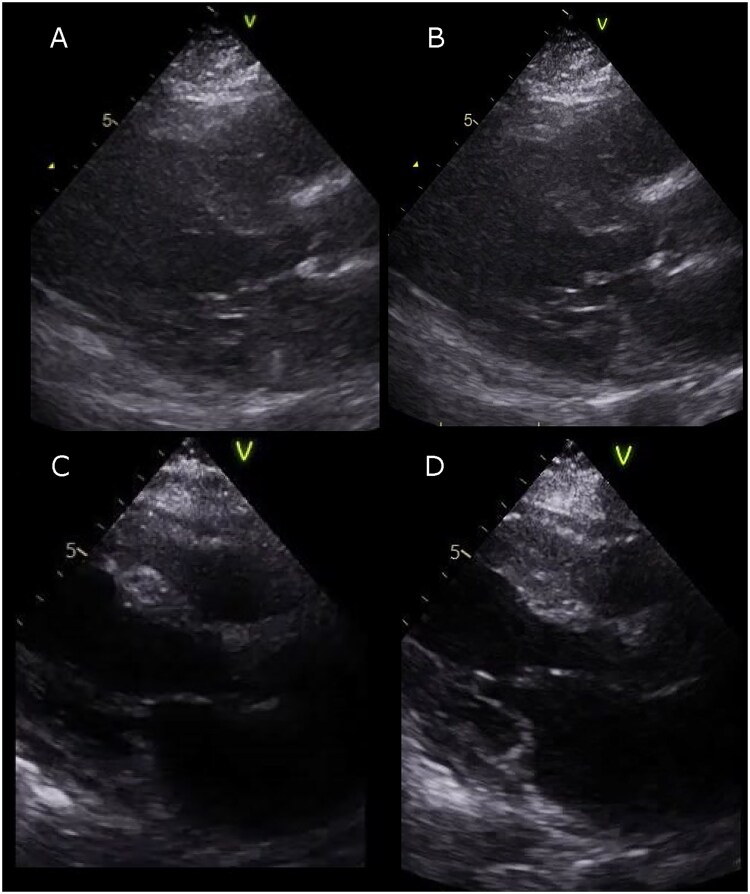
On admission (A: End-systolic, B: End-diastolic) shows severely depressed left ventricular (LV) systolic dysfunction with an LV ejection fraction (LVEF) as low as 38% in transthoracic echocardiography. Finally, LVEF recovered to 54% (C: End-systole, D: End-diastole).
